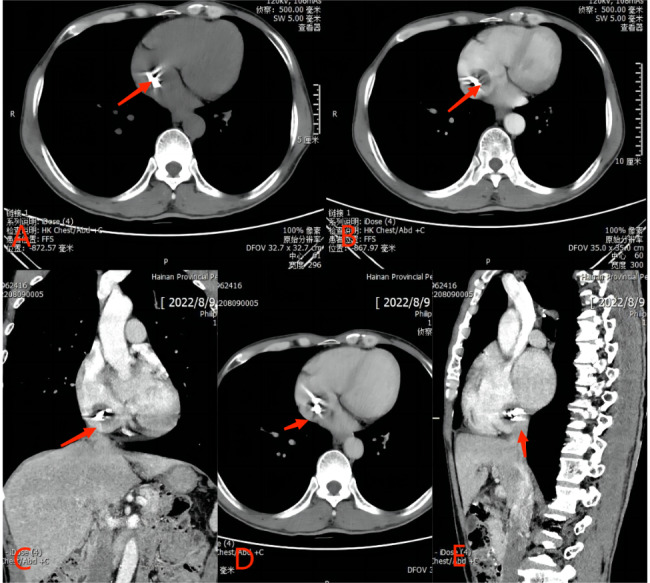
CT examination of hemangioma, (A) Abnormal structure in the right atrium by plain CT (red arrow), (B) Contrast-enhanced arterial phase, (C) Coronal view, (D) Contrast-enhanced venous phase, (E) Sagittal view
